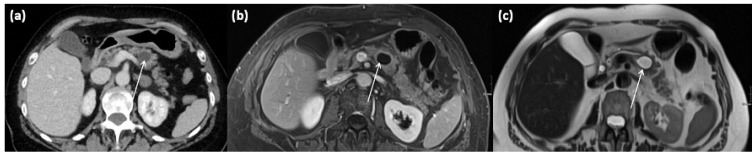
Axial CT in portal venous phase (a) and axial MRI with T1w after gadolinium-based contrast administration (b) as well as T2w native (c) in a 64-year-old female patient. In the pancreatic body, a sharply demarcated, roundly configured, cystic lesion is present, fit to a branch duct IPMN (arrows). There are no main duct dilatation, solid parts, or vegetations. T2w shows a homogenous hyperintense signal.
